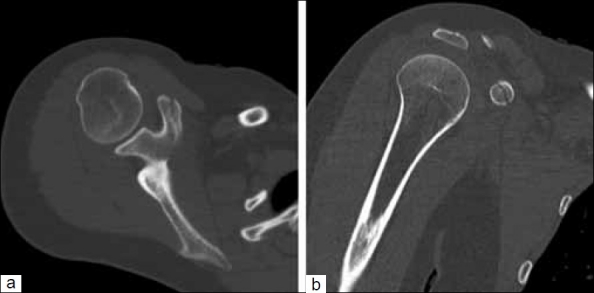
(a) Axial and (b) coronal computed tomography images demonstrate a sagittal plane fracture through the coracoid process
